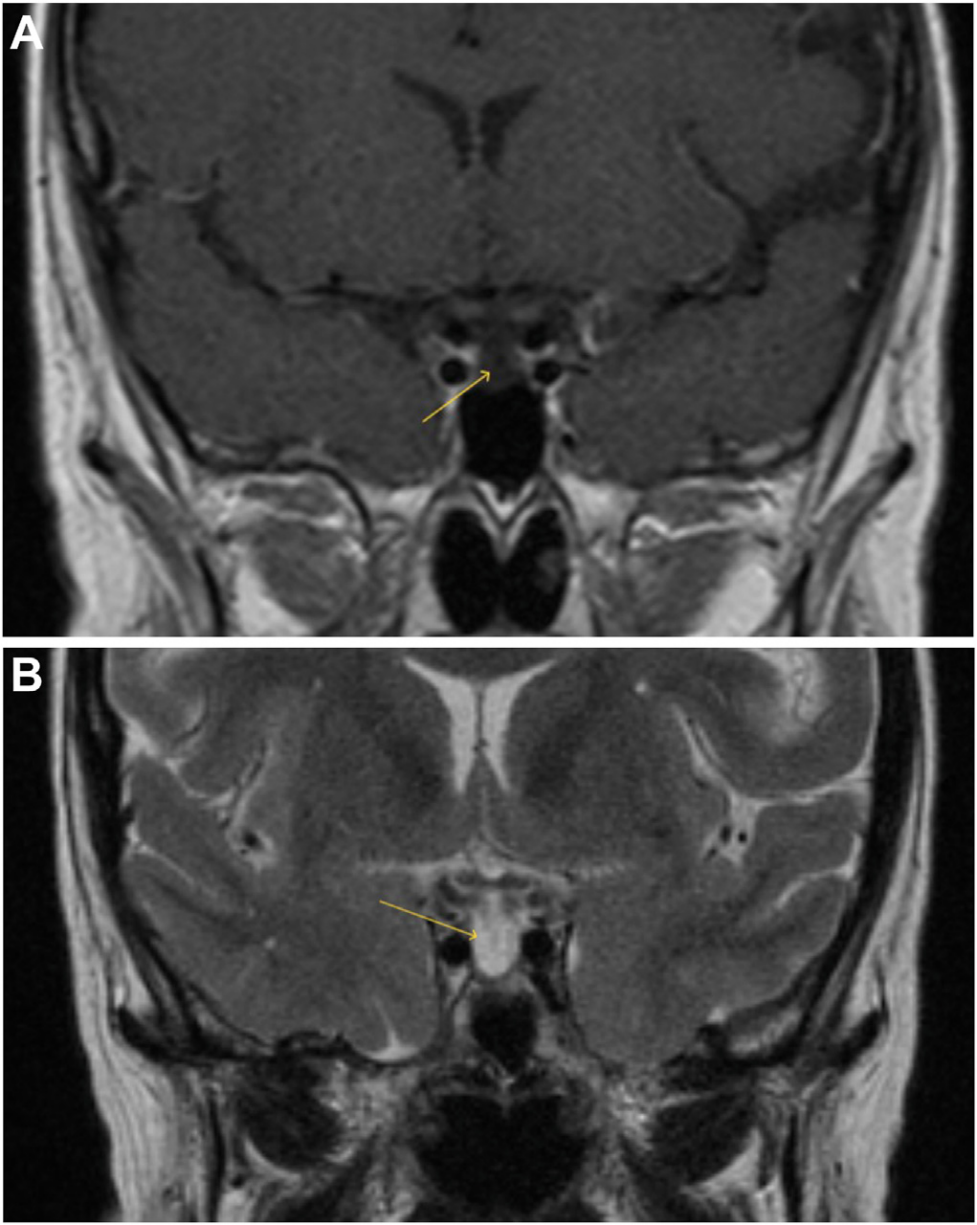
Coronal MRI of the pituitary gland showing empty sella. (A) T1-weighted image demonstrating herniation of the subarachnoid space into the sella turcica (yellow arrow) and a flattened pituitary gland. (B) T2-weighted image highlighting cerebrospinal fluid (CSF) signal within the sella with sellar ballooning (yellow arrow). *MRI* = magnetic resonance imaging.
